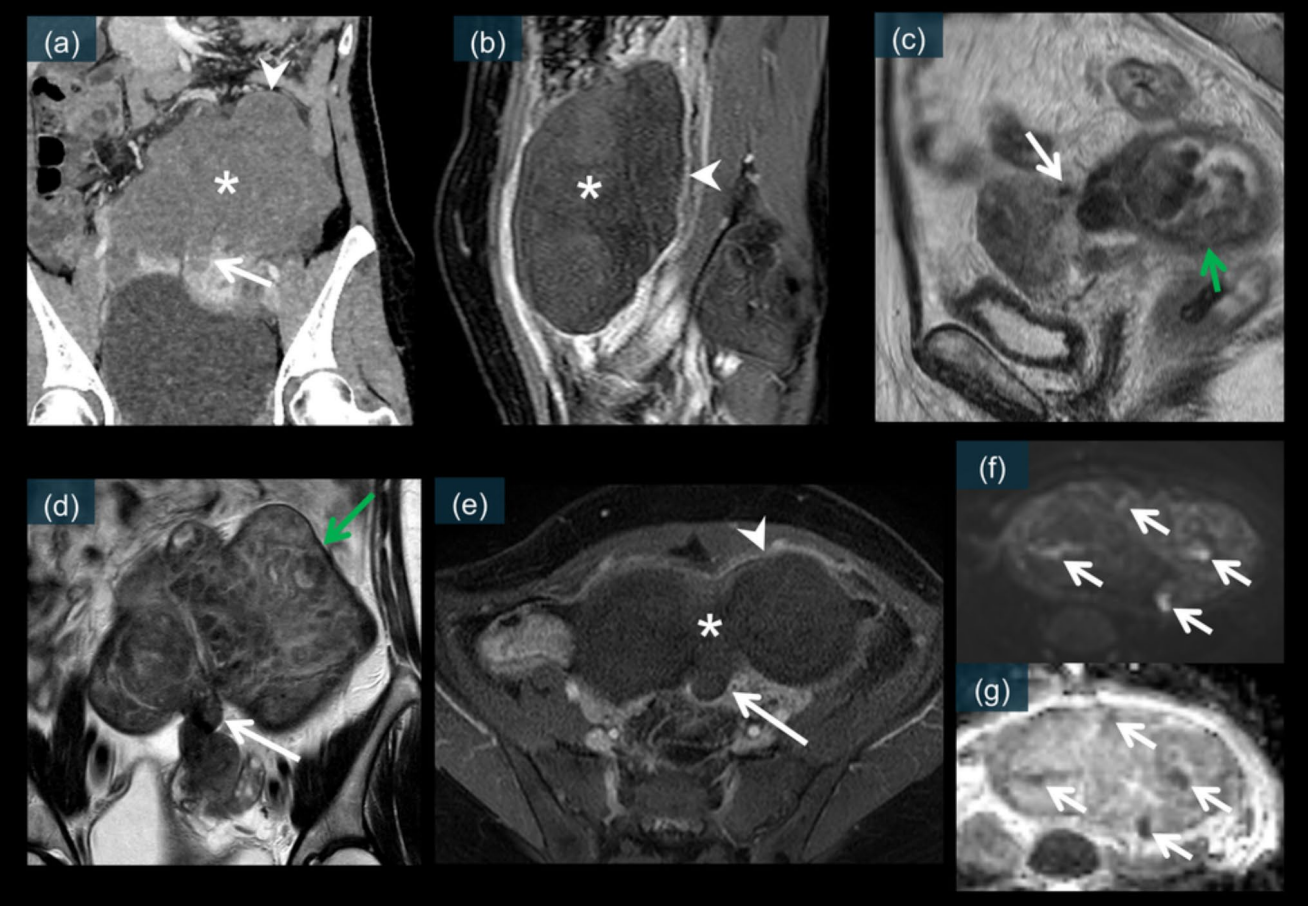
Key diagnostic signs of uterine leiomyoma torsion on cross-sectional imaging. a Contrast-enhanced CT showing the “dark fan sign”—decreased enhancement of the uterine myometrium adjacent to the twisted leiomyoma (white arrow)—and poor internal enhancement of the leiomyoma (white asterisk) with thin peripheral rim enhancement (white arrowhead). b Contrast-enhanced T1-weighted image demonstrating poor internal enhancement of the leiomyoma (white asterisk) with a thin peripheral rim enhancement (white arrowhead). c Sagittal T2-weighted image showing the "bridging vessel sign” (white arrow)—vascular pedicle connecting the leiomyoma to the uterus—and heterogeneous signal intensity within the leiomyoma (green arrow). d Coronal T2-weighted image displaying an enlarged stalk (white arrow) and heterogeneous signal intensity within the leiomyoma (green arrow). e Contrast-enhanced T1-weighted image showing a non-enhancing, wedge-shaped area (white arrow) corresponding to the “dark fan” sign; the mass itself shows no internal enhancement (white asterisk), with only a thin, enhanced peripheral rim (white arrowhead). f Diffusion-weighted image showing high signal intensity (white arrows) within the leiomyoma, indicating restricted diffusion. g ADC map demonstrating corresponding low values (white arrows), confirming ischemic changes
